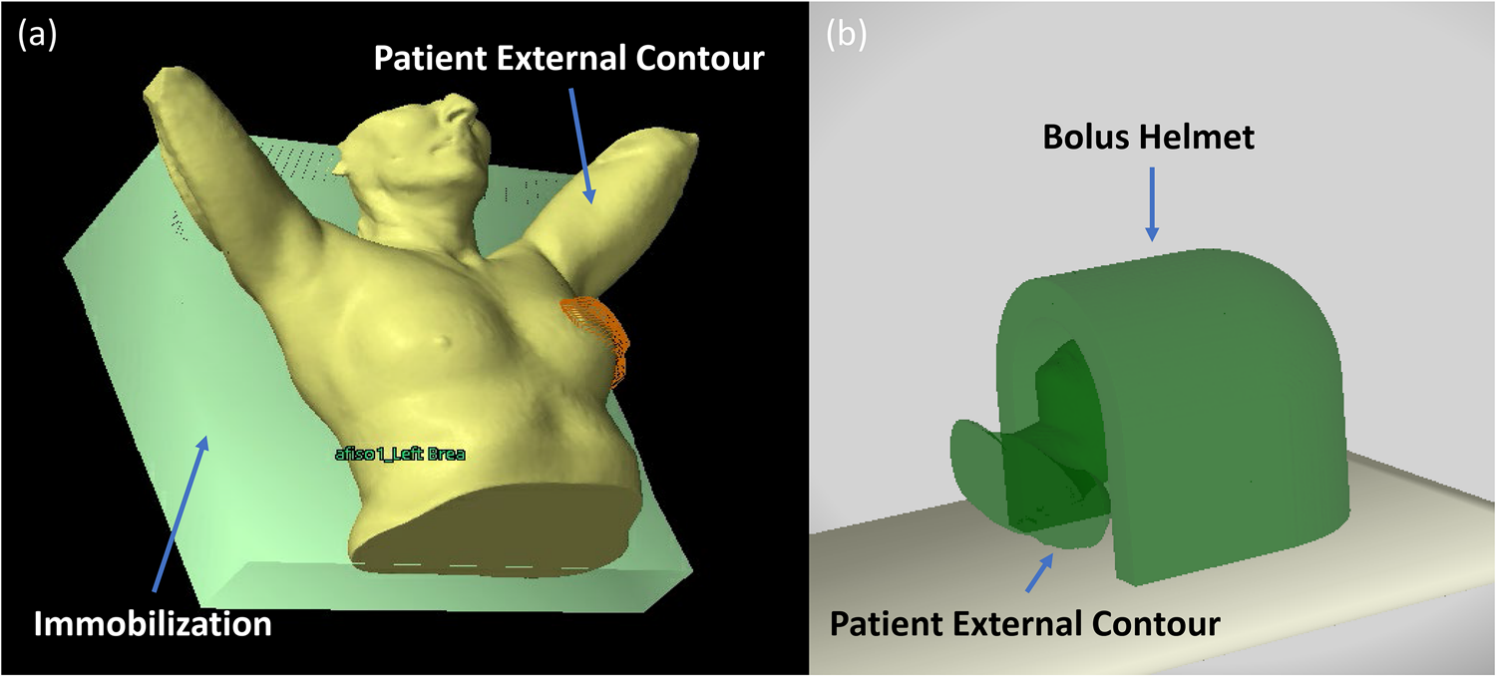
(a) A breast patient external geometry from computed tomography (CT) image contours and coarse contour of the breast board immobilization device. (b) A patient external contour and bolus helmet structure from CT image contours
